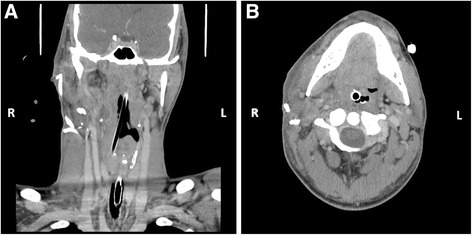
Computed tomographic scans of the neck showing edema and copious liquid in the cervical-facial spaces, exerting a mass effect over the airways with complete obliteration at the soft palate level. a Coronal section of the head and neck showing marked swelling of the right parotid and mandibulopharyngeal spaces with left deviation of the airway. b Axial section of the head at the level of the oropharynx showing marked edema of the right tonsil and soft palate with compromise of airway patency. *L* Left, *R* Right
